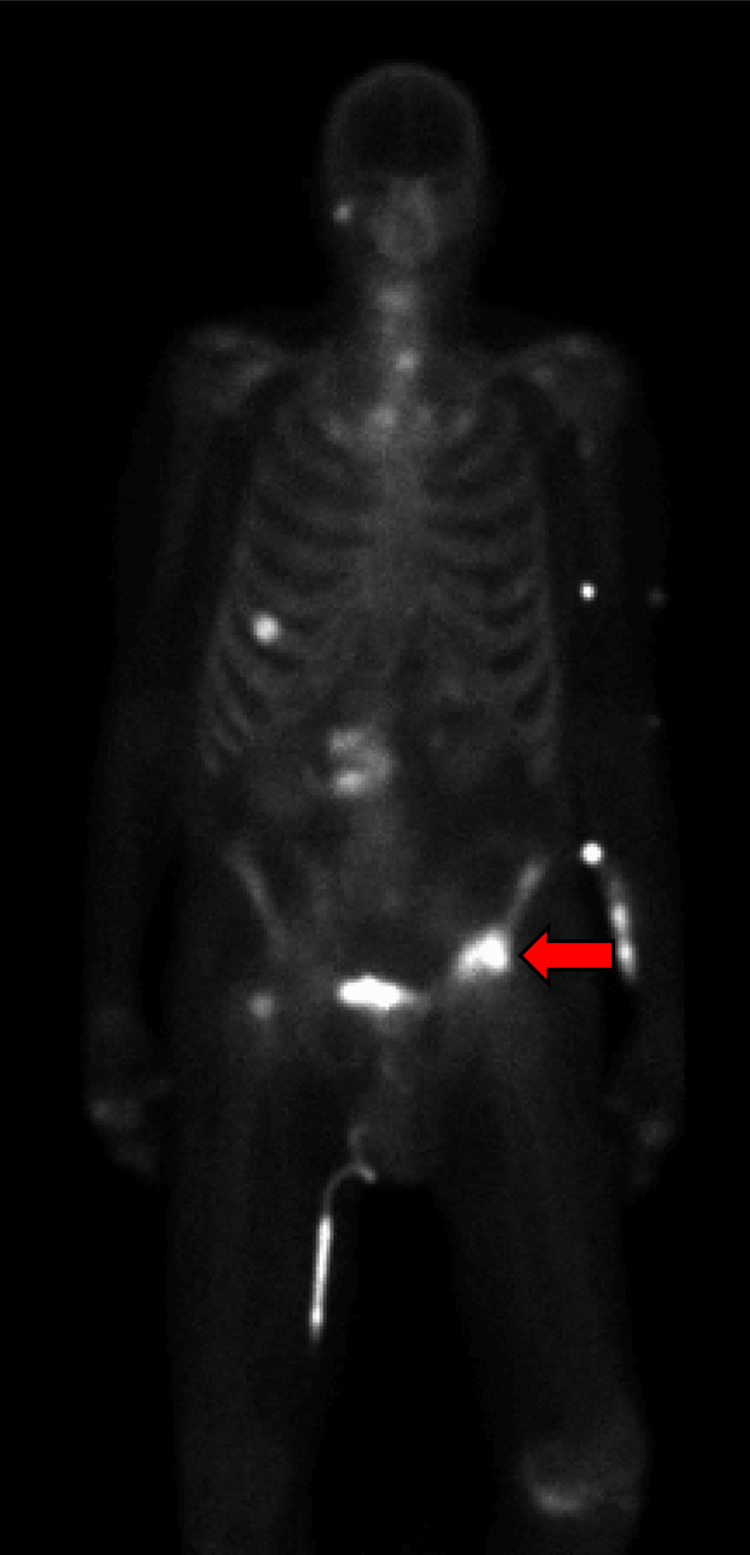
Nuclear medicine (NM) bone scan demonstrating radiotracer uptake in the right temporo-occipital skull, left proximal and mid-humerus, right eighth anterior rib, left hemipelvis, right femoral neck, sacrum, and segments of the cervical, thoracic, and lumbar vertebrae The lesion initially seen on CT and MRI in the left hemipelvis notably shows the most significant uptake among bony lesions.
